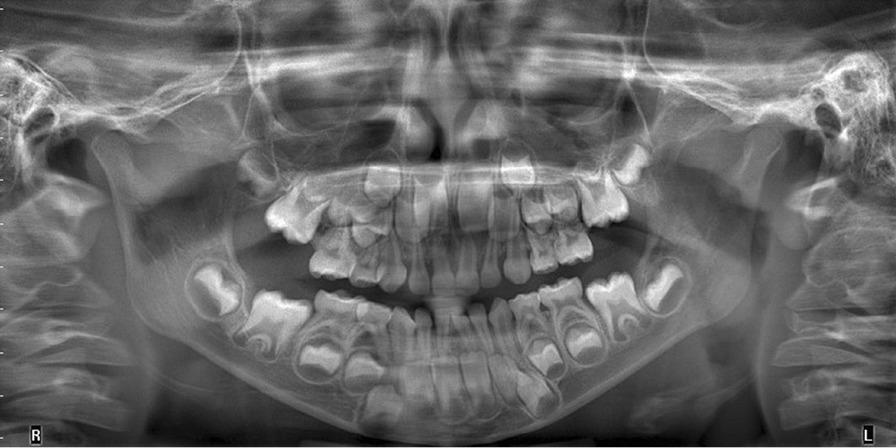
Panoramic X-ray at initial examination failed to demonstrate the lesion or any bone involvement/destruction
